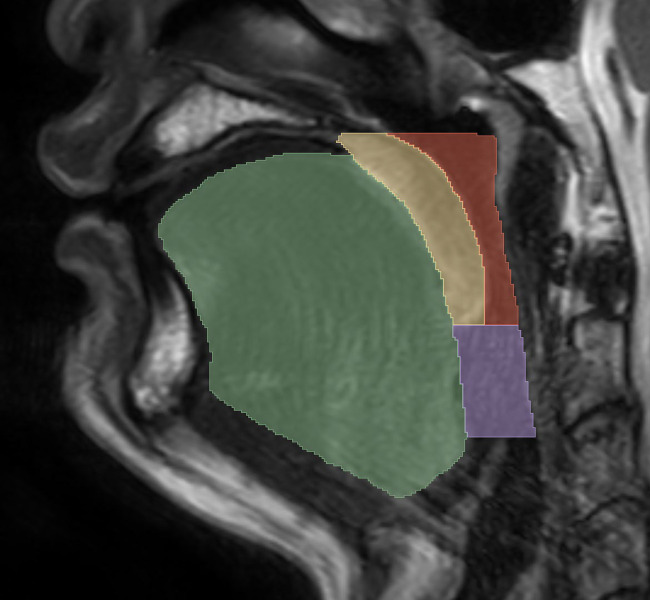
The sagittal MRI image showing the tongue (green), soft palate (yellow), retropalatal airway (red), and retroglossal airway (purple). MRI = magnetic resonance imaging.
